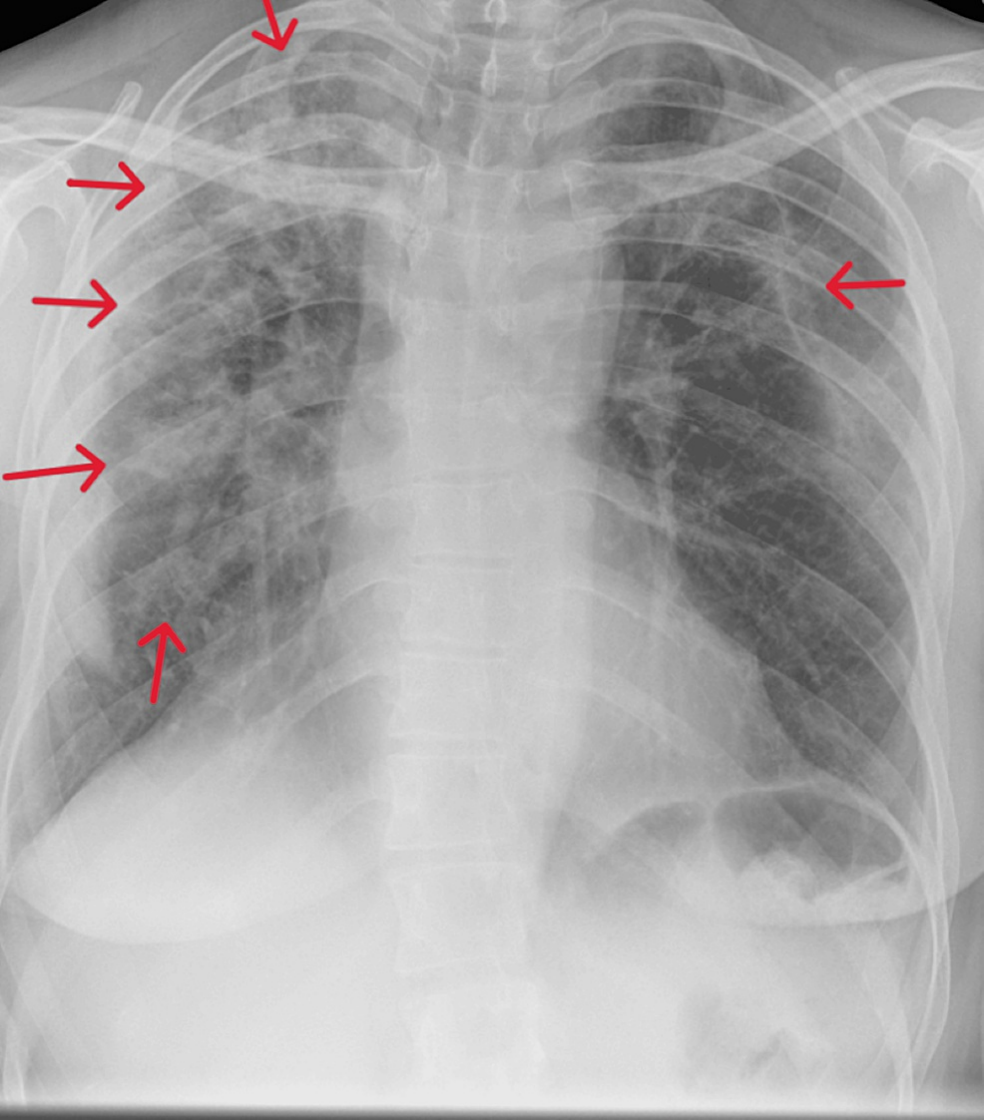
Repeat chest X-ray was conducted two weeks after the patient's admission. Arrows indicate worsened bilateral opacification compared to Figure 2.
